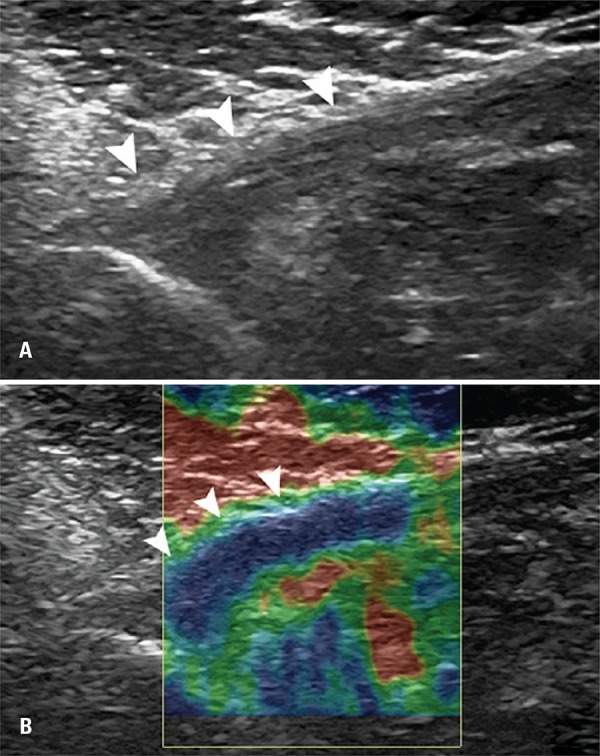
Ultrasound assessment of pectoralis major muscle tendon with signs of tendinous injury. (A) Thickened tendon and reduced ecogenicity – tendinous injury (arrowheads). (B) Elastography assessment showing stiff pattern (blue)
